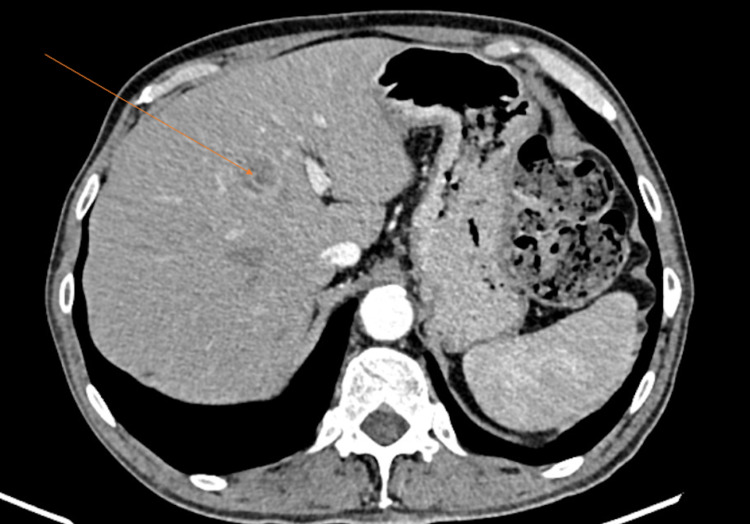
Axial section of triphasic CT of the abdomen. The arrow depicts a hypoenhancing, space-occupying lesion in the segment IV of the liver with peripheral enhancement on the portal phase.
